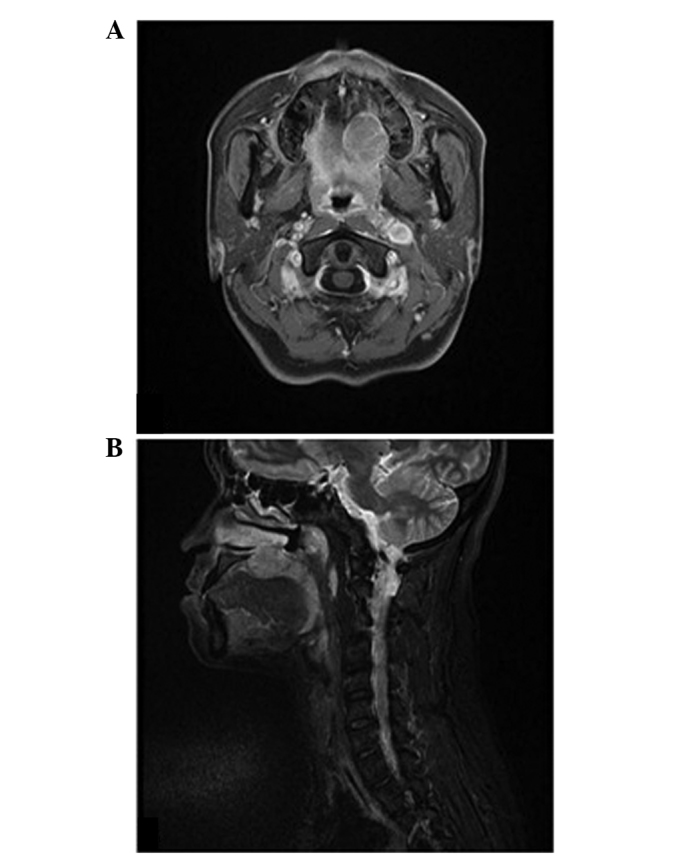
(A) Axial and (B) sagittal magnetic resonance images show a mass of 26 × 24 × 17 mm in size, located on the left hard palate with invasion into the nasal cavity.
